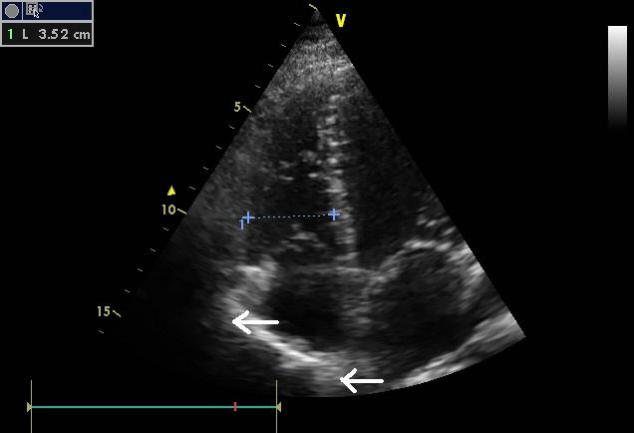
Postoperative echocardiography image showing the second atrial auricle (superior arrow), as well as the indigenous auricle on the bottom of the image (inferior arrow).
